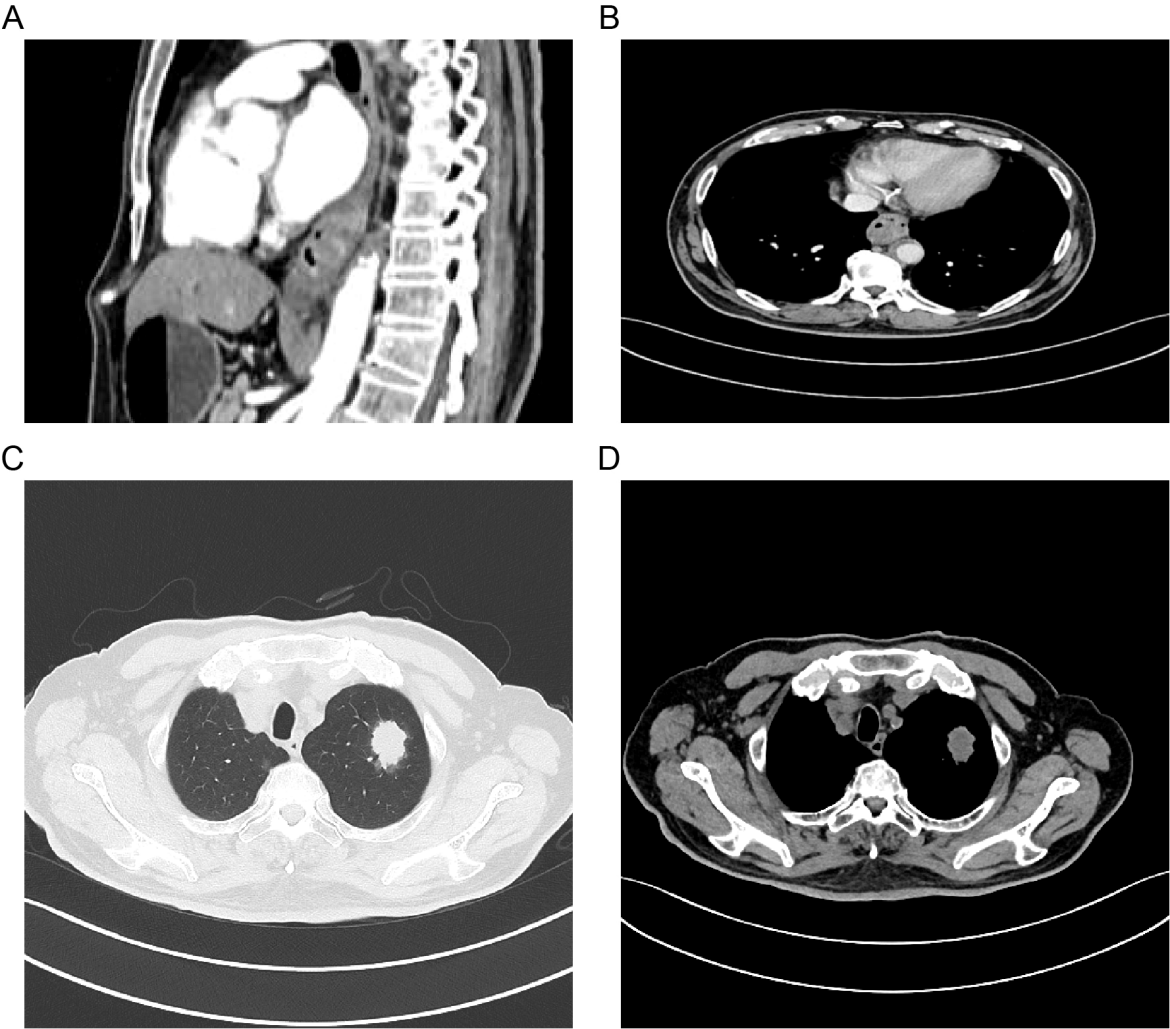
Abdominal (A, B) and thoracic (C, D) contrast-enhanced CT images.
